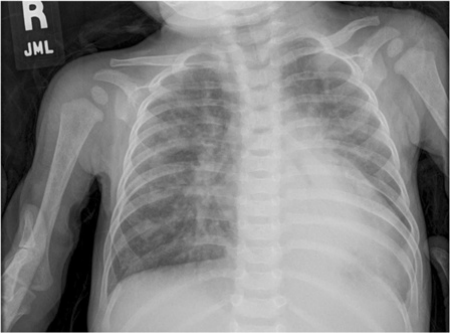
The chest x-ray, viewed in the antero-posterior projection, from a typical patient with ventricular septal defect shows cardiomegaly, increased pulmonary vascular markings, and atelectasis of the left lower lobe.
